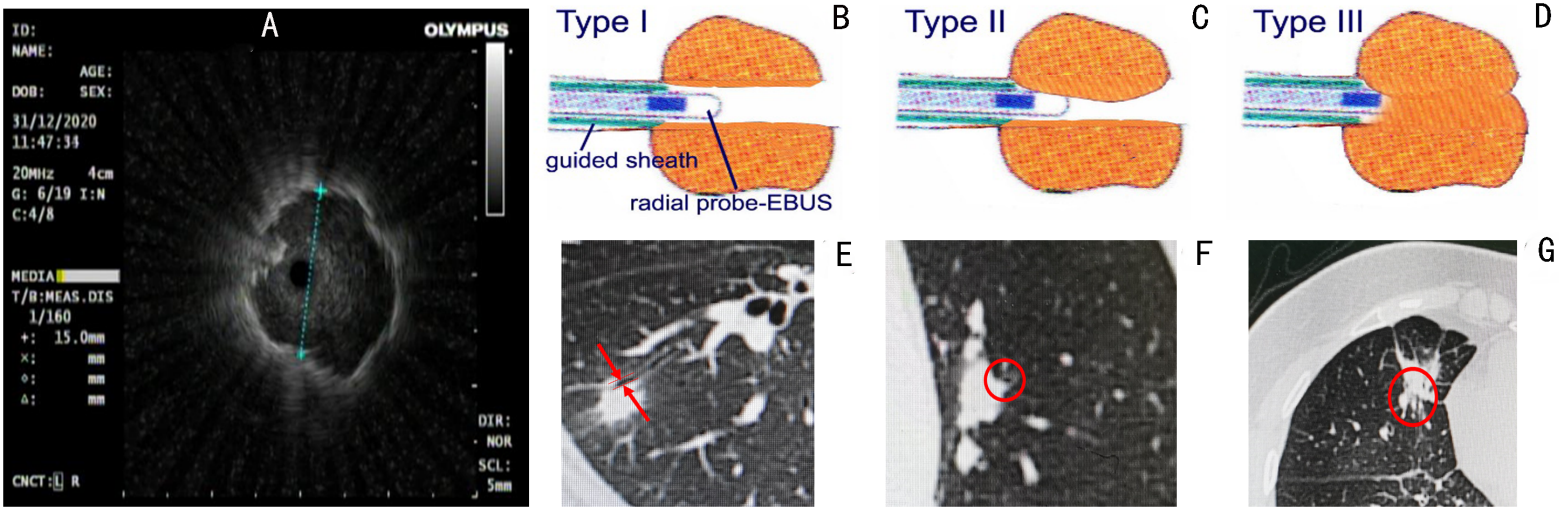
Radial probe endobronchial ultrasonography (EBUS) view of a peripheral target nodule where an EBUS probe is located within the target lesion (A). We categorized the probe resistance in each case into three types I to III according to the different resistance of the probe to pass through the lesion. In type I, EBUS probe can pass through the lesion without resistance (B) where the bronchus in relation to the lesion was >1.4 mm in diameter (E). In type II and type III, the corresponding bronchi were ≤ 1.4 mm in diameter (F, G); for type II, EBUS probe could pass through the lesion with moderate resistance (C), and for type III, EBUS probe could not pass through the lesion with high resistance (D).
